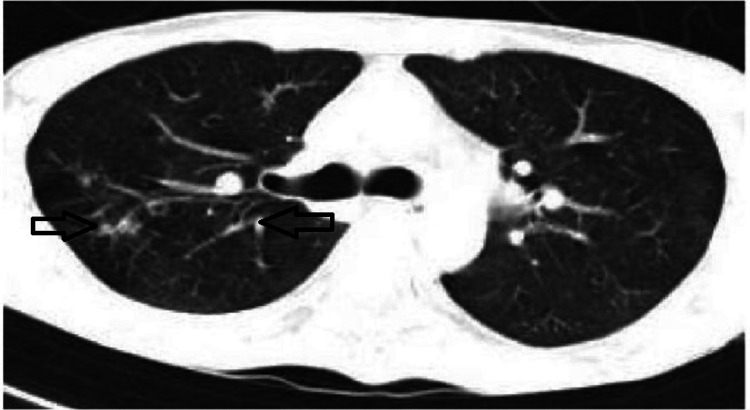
HRCT thorax revealed ground glass opacity. HRCT, high-resolution computed tomography
